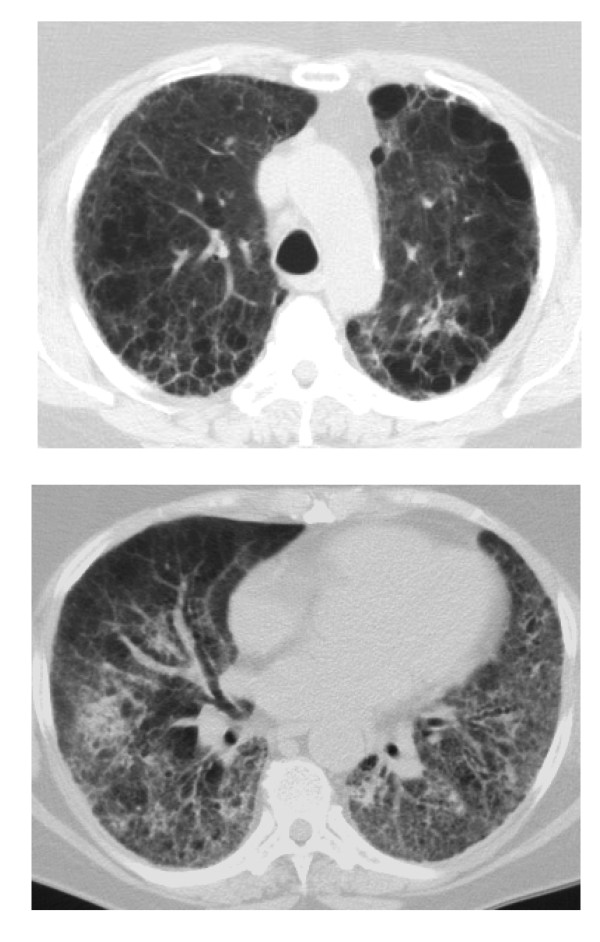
Chest computed tomogram from a patient with pulmonary fibrosis and an emphysema score of 12. The upper panel shows advanced predominantly centrilobular emphysema at the level of the aortic arch. The lower panel shows mild ground glass opacities, substantial reticular opacities, and advanced traction bronchiectasis at the lung bases. Surgical lung biopsy in this patient showed usual interstitial pneumonia (UIP).
